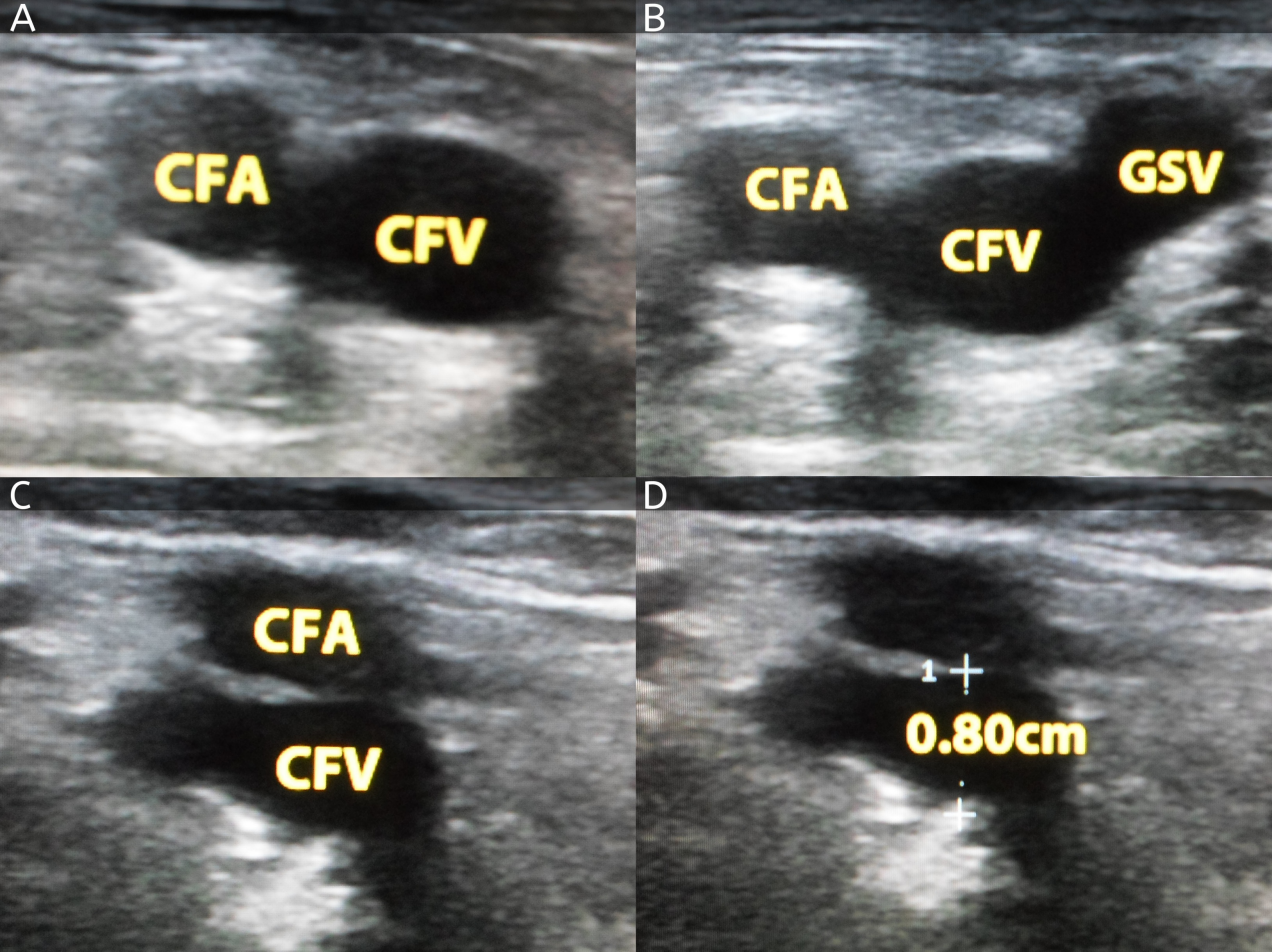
Ultrasonographic images for various measurements A. Ultrasound image of common femoral artery (CFA) and common femoral vein (CFV) taken at inguinal ligament transversely. B. The great saphenous vein (GSV) joining the common femoral vein. C. Image taken at distal site of CFV where the GSV disappears (the point where FVD was measured). D. FVD was measured anteroposteriorly at the center.
